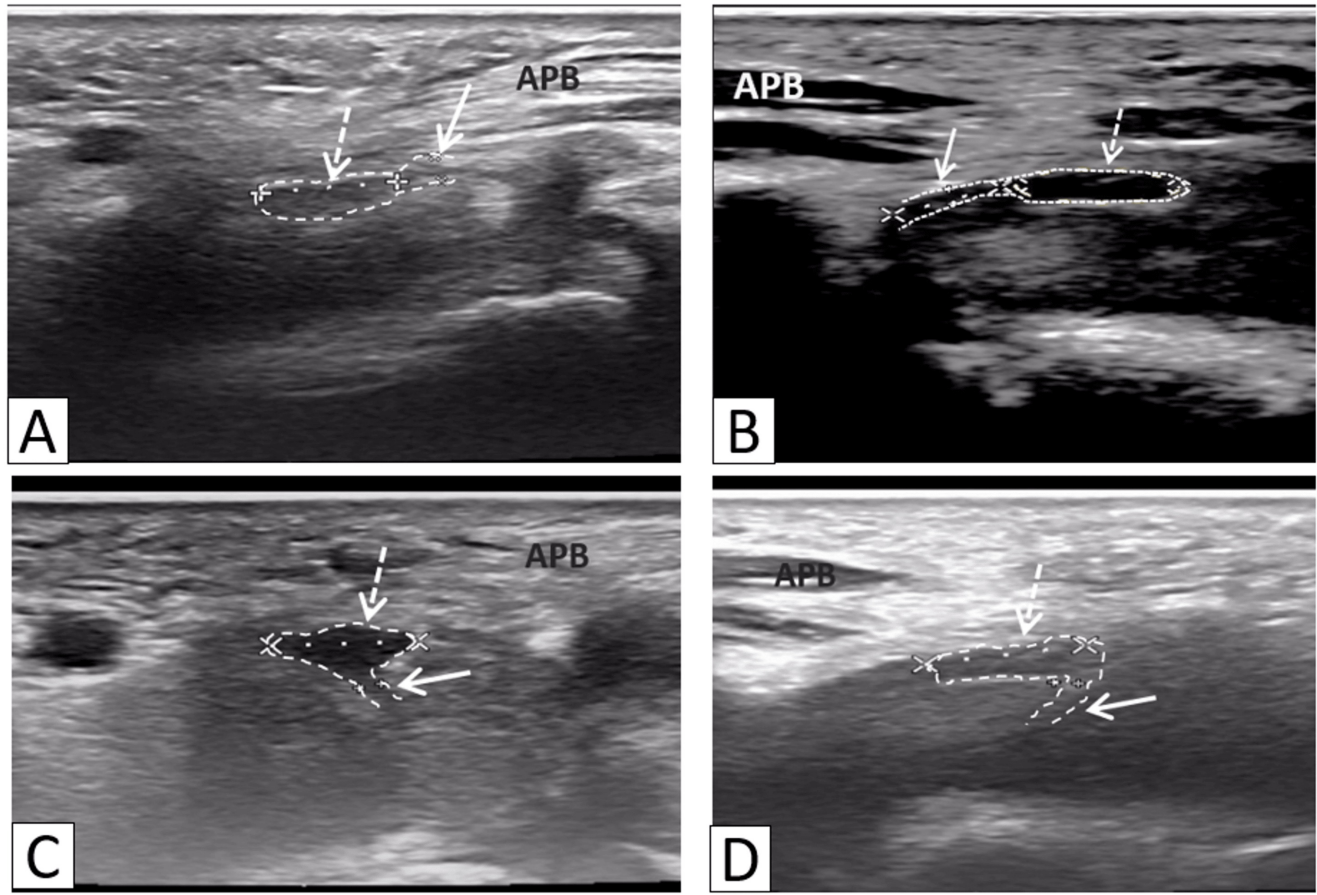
Transverse 18–4-MHz ultrasound imaging of the median nerve (dashed arrow) at the level of the origin of the left (A) and right (B) recurrent motor branch (solid arrow), and at the level of the origin of the left (C) and right (D) branches to the first and second lumbrical muscles (solid arrow). The ultrasound probe is positioned over the thenar region, perpendicular to the first metacarpal bone. This ultrasound image demonstrates an enlarged recurrent motor branch (A, solid arrow) arising from the radial division of the median nerve (dashed arrow).
